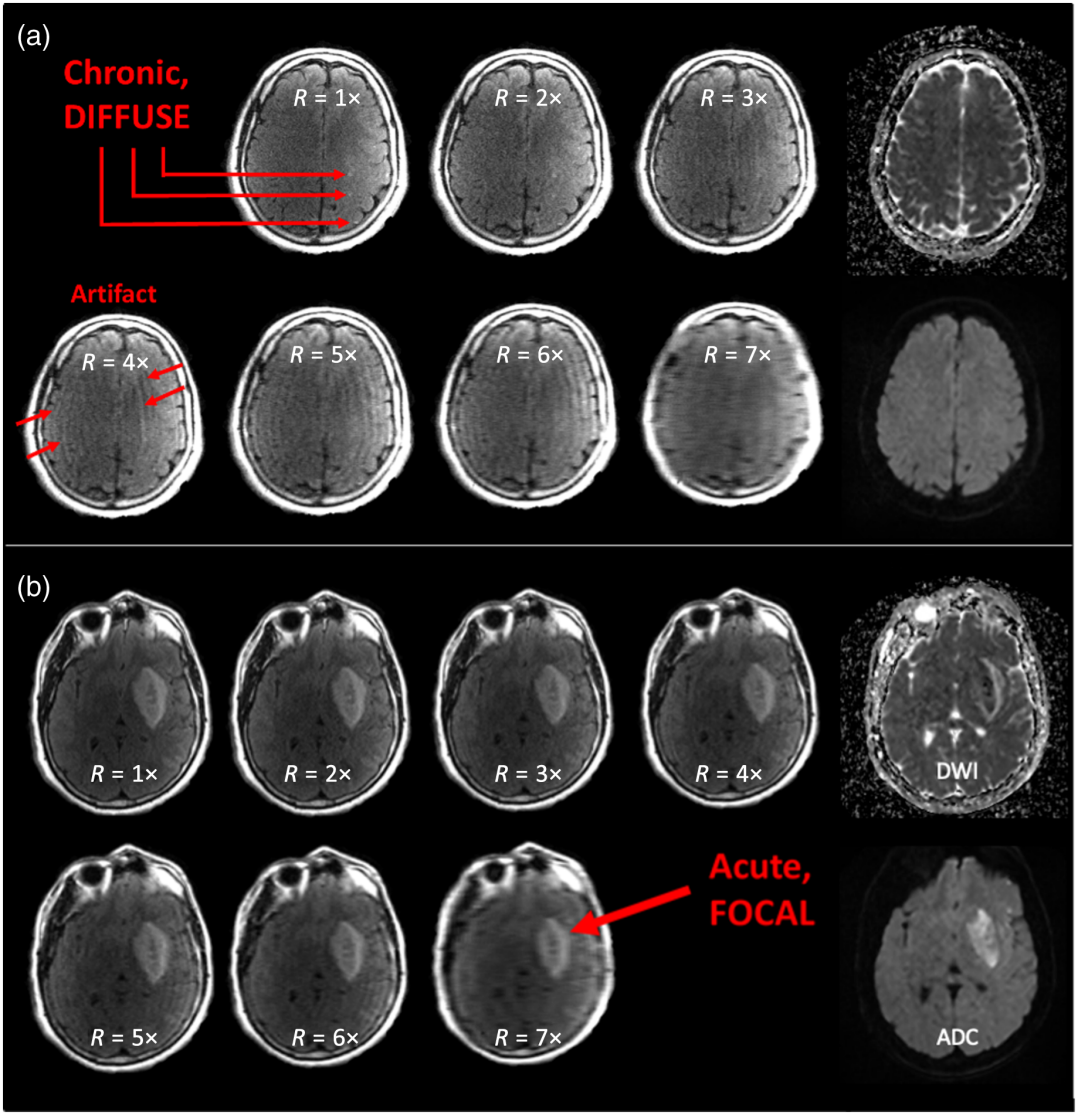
2D T2 fluid-attenuated inversion recovery (FLAIR) image slices from an accelerated MR image dataset corresponding to a representative acute ischemic stroke (AIS) patient. Unique anatomical slices show (a) chronic ischemic lesions and (b) AIS, as visualized in images with R=1× (fully sampled reference) and R=2 to 7× (undersampled images). Undersampling followed by compressed sensing reconstruction resulted in variations in image quality. Matched slices from the DWI series and ADC map are also shown for comparison.
